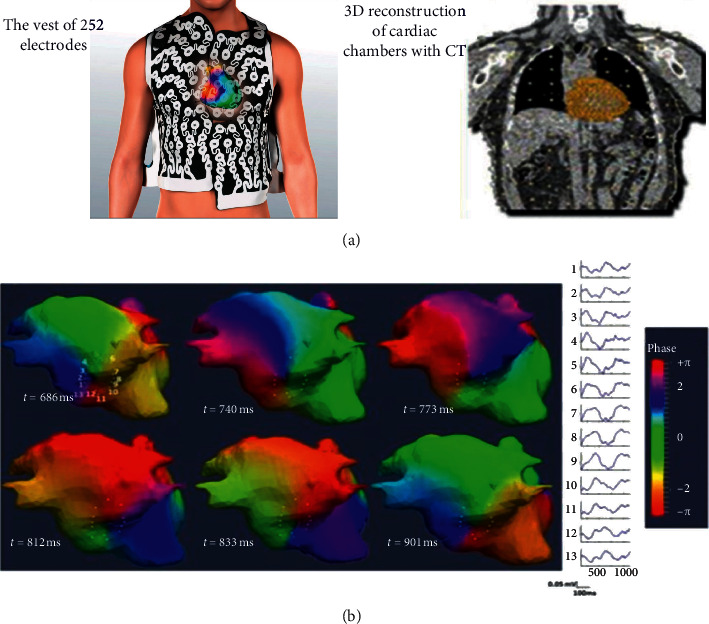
Noninvasive body surface mapping. (a) The vest of 252 electrodes and the three-dimensional reconstruction of cardiac chambers with the computed tomography. The voltage at each point of the torso, taken from the 252 electrodes of the vest, is depicted with virtual electrograms on the surface of the atria. (b) Isochronous maps showing the rotational activity near the antrum of the right inferior PV.
